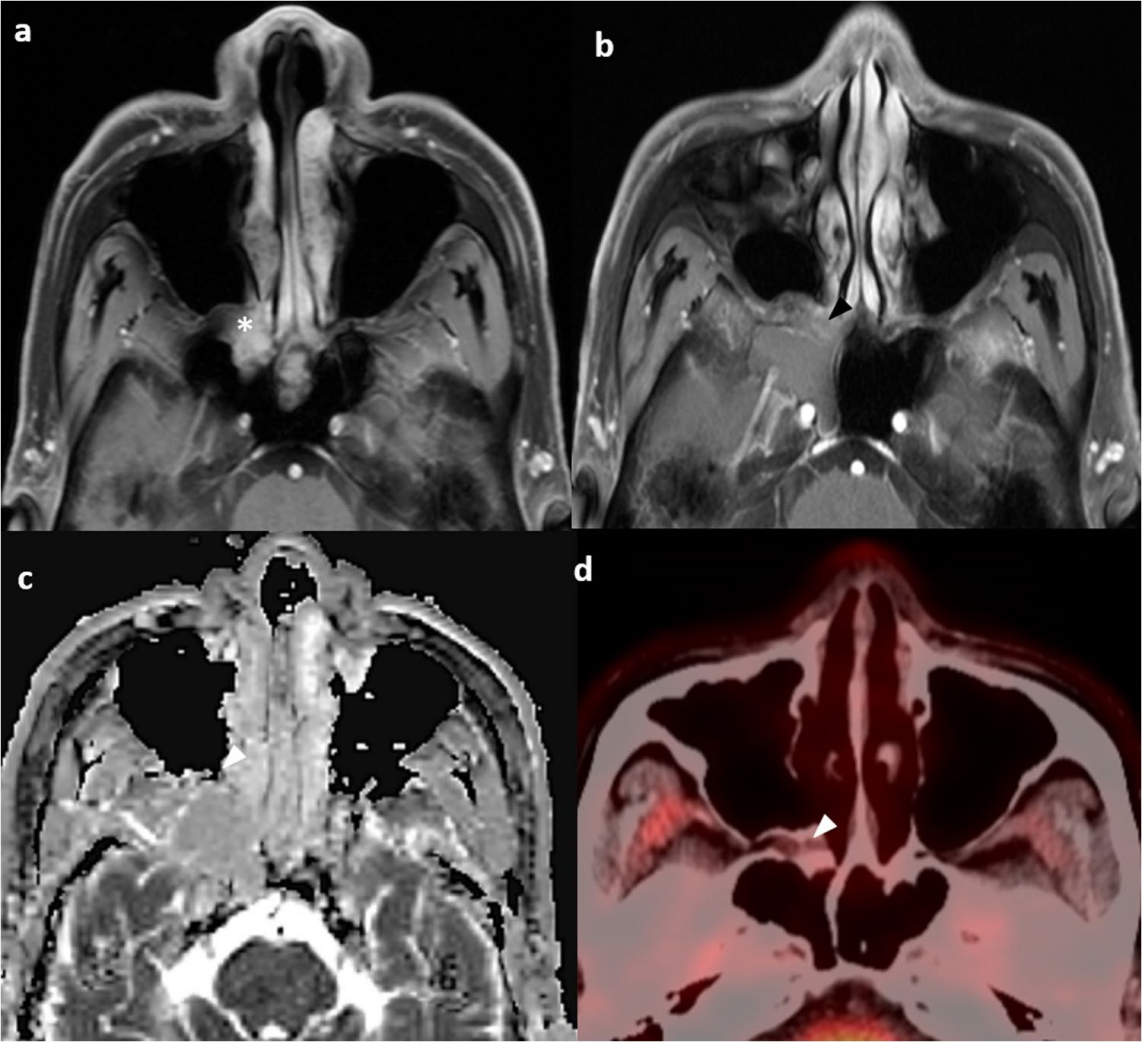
Scar tissue in a 65-year-old man who had NPC (clinical stage T3N1M0) involving the right pterygopalatine fossa completed combined chemoirradiation in 2022. Post-treatment endoscopy revealed persistent tumor and therefore stereotactic radiotherapy was given. Axial T1-weighted post-contrast image with fat suppression shows the enhancing soft tissue at the right pterygopalatine fossa with local expansion (asterisk) suggestive of tumor (a). Axial T1-weighted post-contrast image with fat suppression performed at 18 months upon treatment completion shows interval shrinkage of the previous noted tumor at right pterygopalatine fossa but with persistent mildly enhancing soft tissue thickening (arrowhead) equivocal for residual tumor or scar (b). ADC map of the diffusion-weighted imaging performed at 18 months upon treatment completion showed no restricted diffusion in the right pterygopalatine fossa (arrowhead) (c). Axial fusion FDG-PET/CT at 18 months post-treatment depicts the right pterygopalatine fossa soft tissue to be non-FDG avid (d). The original tumor at the right nasopharynx has resolved (not shown). Findings are suggestive of scar tissue without viable tumor
